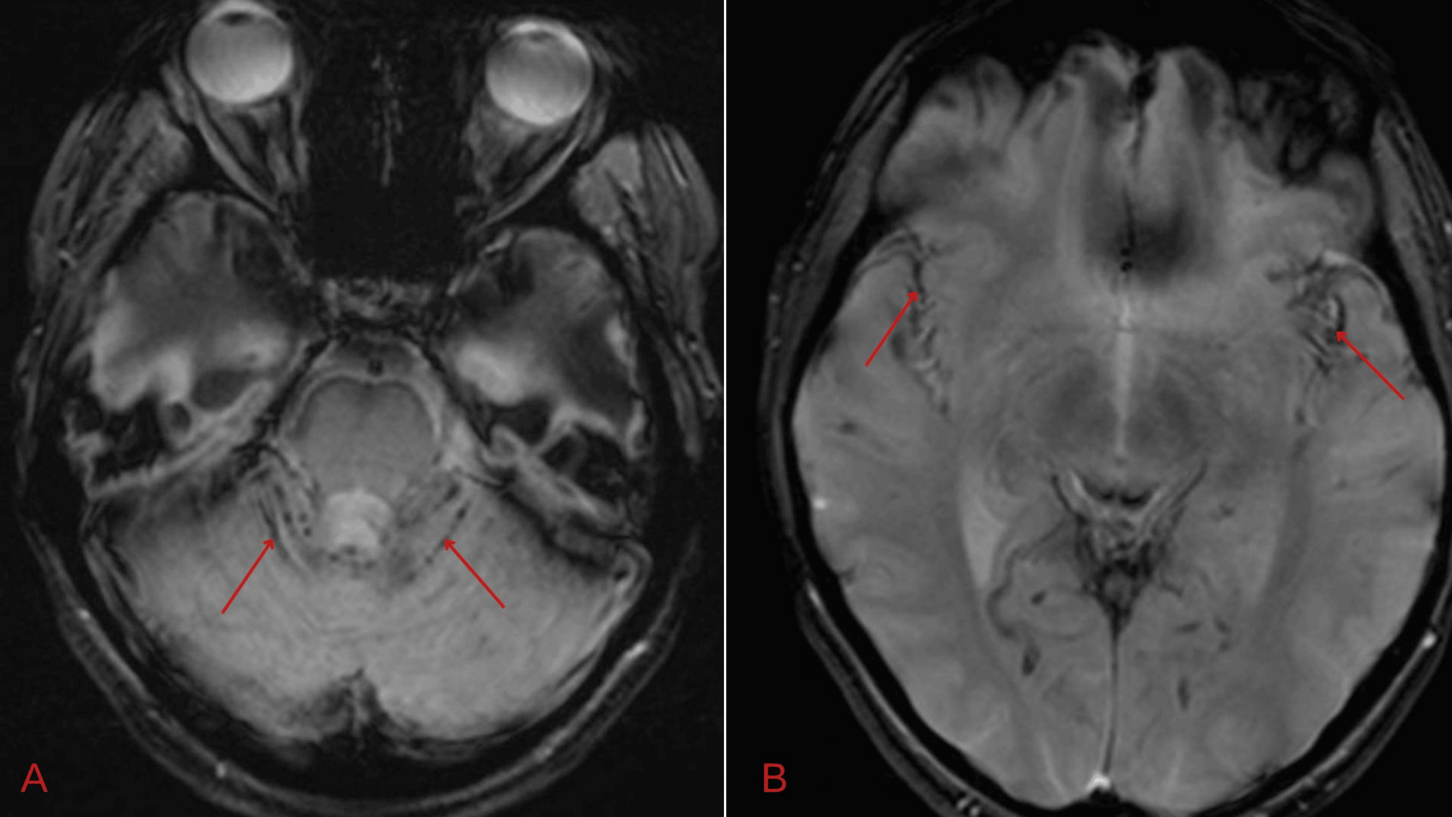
MRI brain axial Gradient Echo T2 sequence revealing superficial siderosis in the posterior fossa (A) and Sylvian fissures (B). MRI, magnetic resonance imaging
